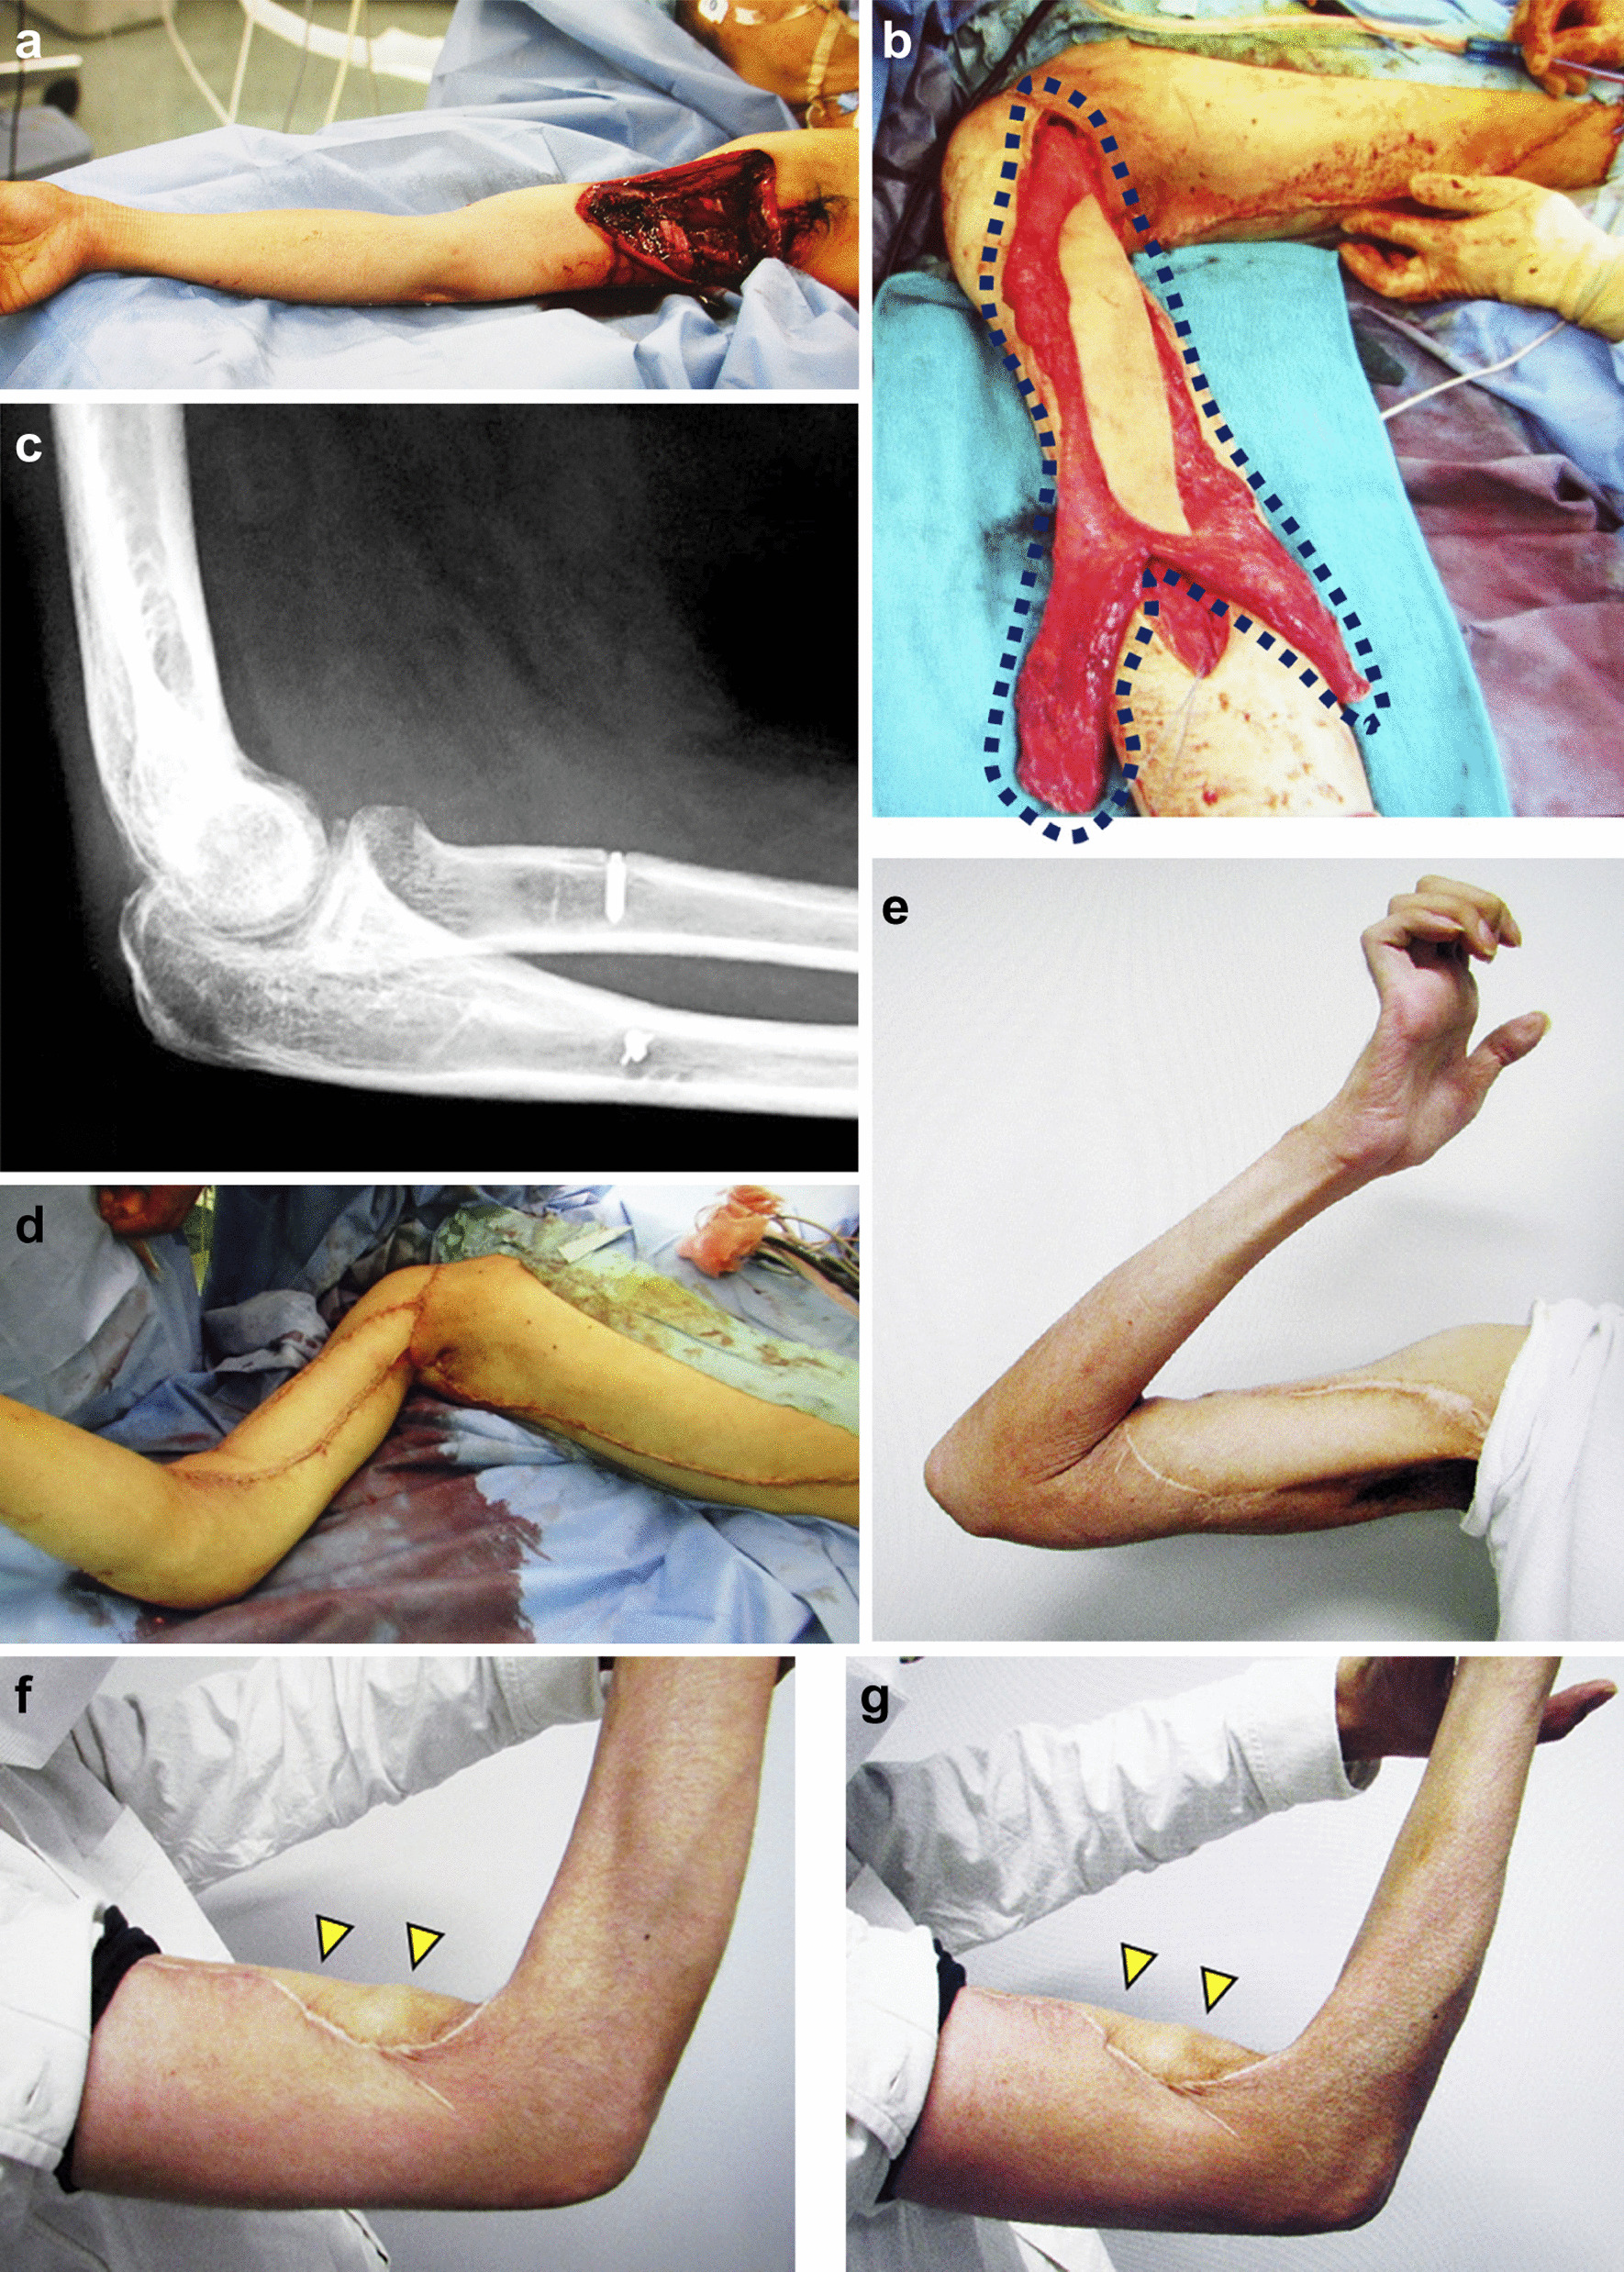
Case 1. a There was severe damage to the musculocutaneous nerve, and a primary suture or graft was deemed impossible. b The distal muscle flap of the transferred llatissimus dorsi muscle was divided into two. c Simple X-ray imaging shows fixation to the radial and ulnar tuberosities with GII^®^ anchors. d Elbow flexion and soft tissue were simultaneously reconstructed with a latissimus dorsi musculocutaneous flap. e Elbow’s active range of motion was 10–145°. f Forearm pronation was performed with extension resistance. Active contraction of the latissimus dorsi muscle with elbow flexion was confirmed (Yellow triangles indicate latissimus dorsi muscle). g Active contraction of the latissimus dorsi muscle was confirmed similarly to forearm supination (Yellow triangles indicate latissimus dorsi muscle)
